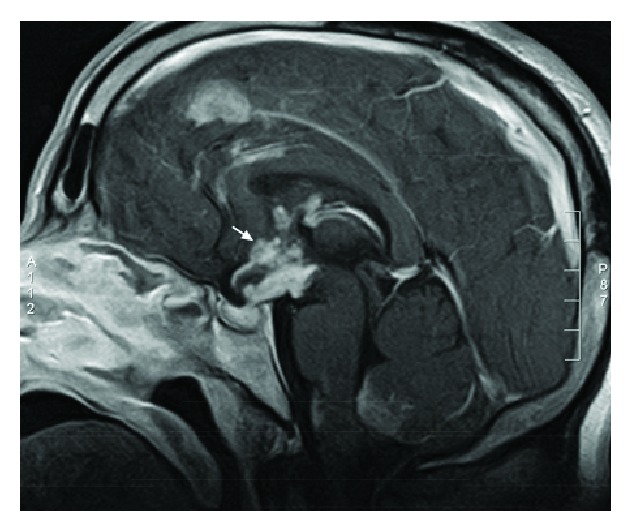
Brain magnetic resonance image showing neurosarcoidosis involving the hypothalamus and pituitary.
